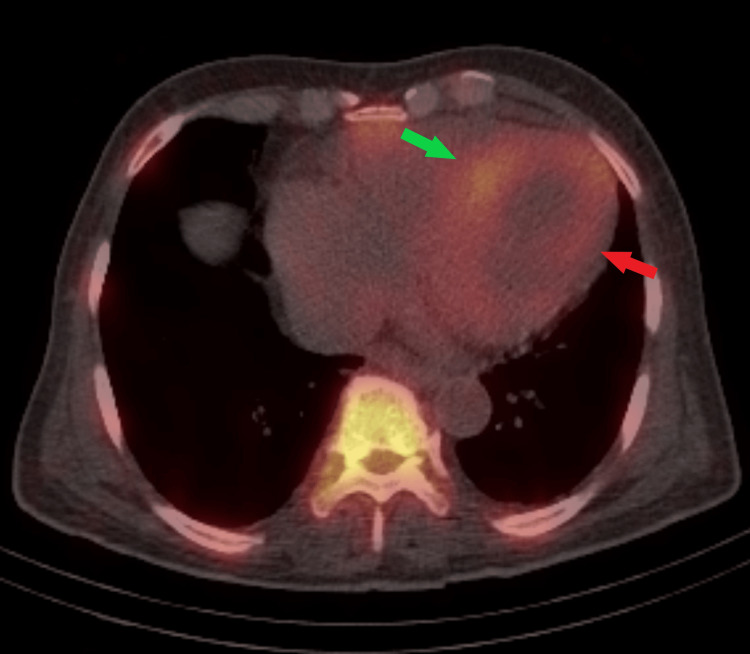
Fused SPECT-CT images of the chest demonstrated diffuse left ventricular myocardial uptake (red arrow) with a focal increase on top of diffuse increased uptake localized to the interventricular septum (green arrow), which appeared similar to the ribs uptake
